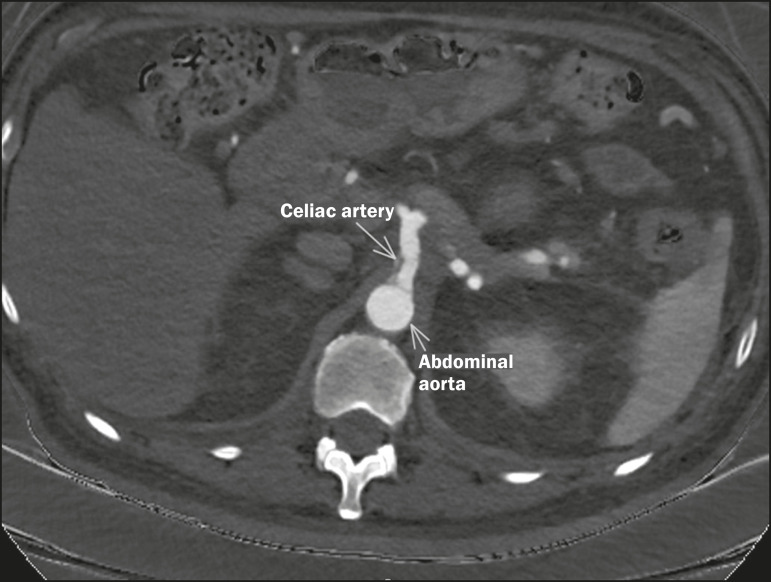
Axial contrast-enhanced CT scan of the abdomen, in the arterial
